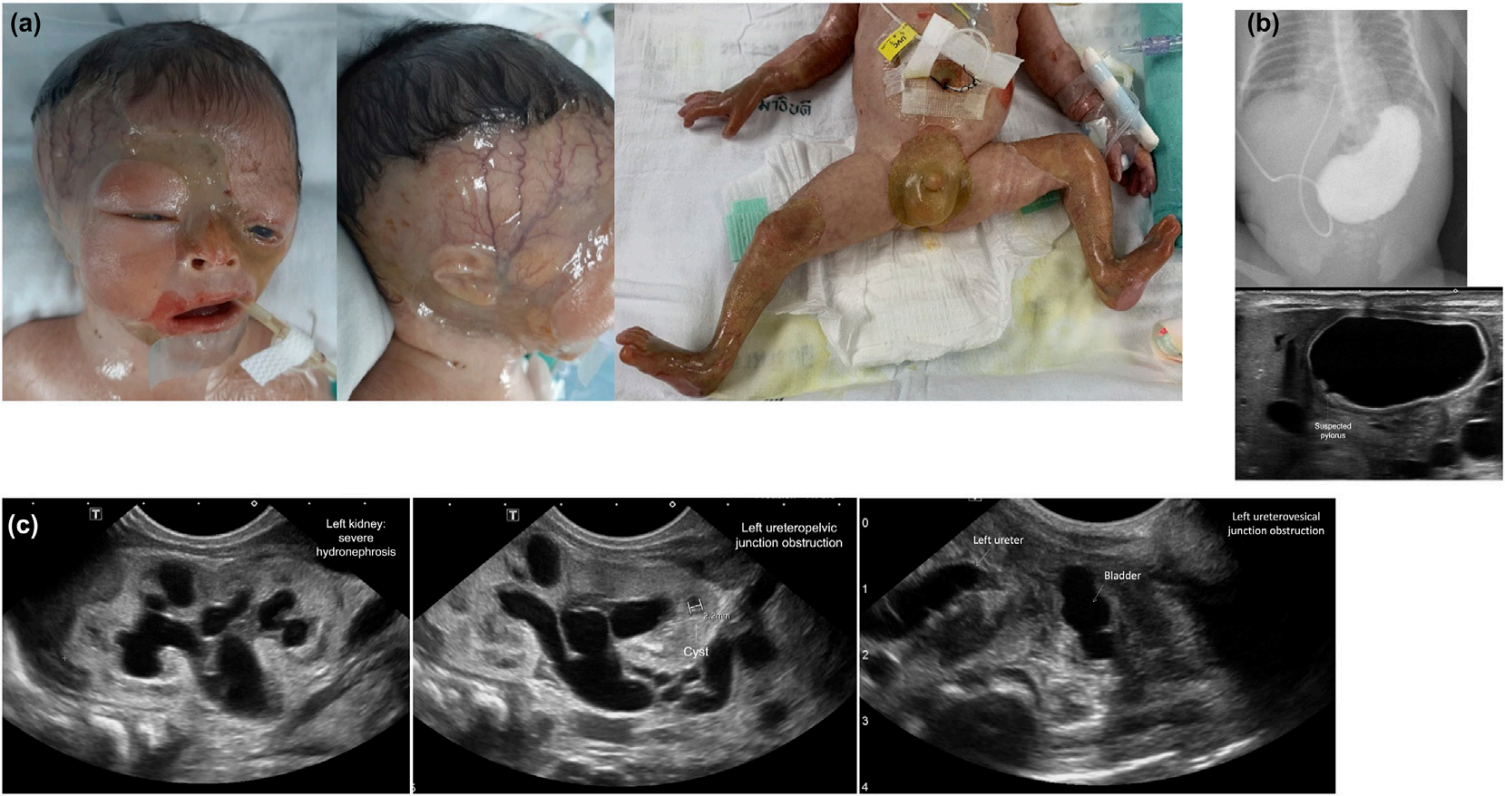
Characteristics of skin involvement and other abnormalities of Patient 4. (A) Skin involvement: marked complete absence of skin over the parietotemporal region of the scalp and extending downward to the interorbital and midfacial areas including the nasal region; perineal area; upper and lower extremities including thighs, legs, feet, arms, forearms, and hands; bilateral cloudy cornea, ectropion of the left lower eyelid and dysplastic ears. (B) Pyloric atresia: an upper gastrointestinal study showing a gastric outlet obstruction and abdominal ultrasound showing large air-filled stomach. (C) Abdominal ultrasound demonstrating multi-leveled urinary tract obstructions: severe hydronephrosis of the left kidney (far left); left ureteropelvic junction obstruction (middle); and a small and collapsed urinary bladder and markedly dilated left ureter, signifying a vesicoureteric junction obstruction (far right). Similar findings were observed on the right kidney and ureter (data not shown).
